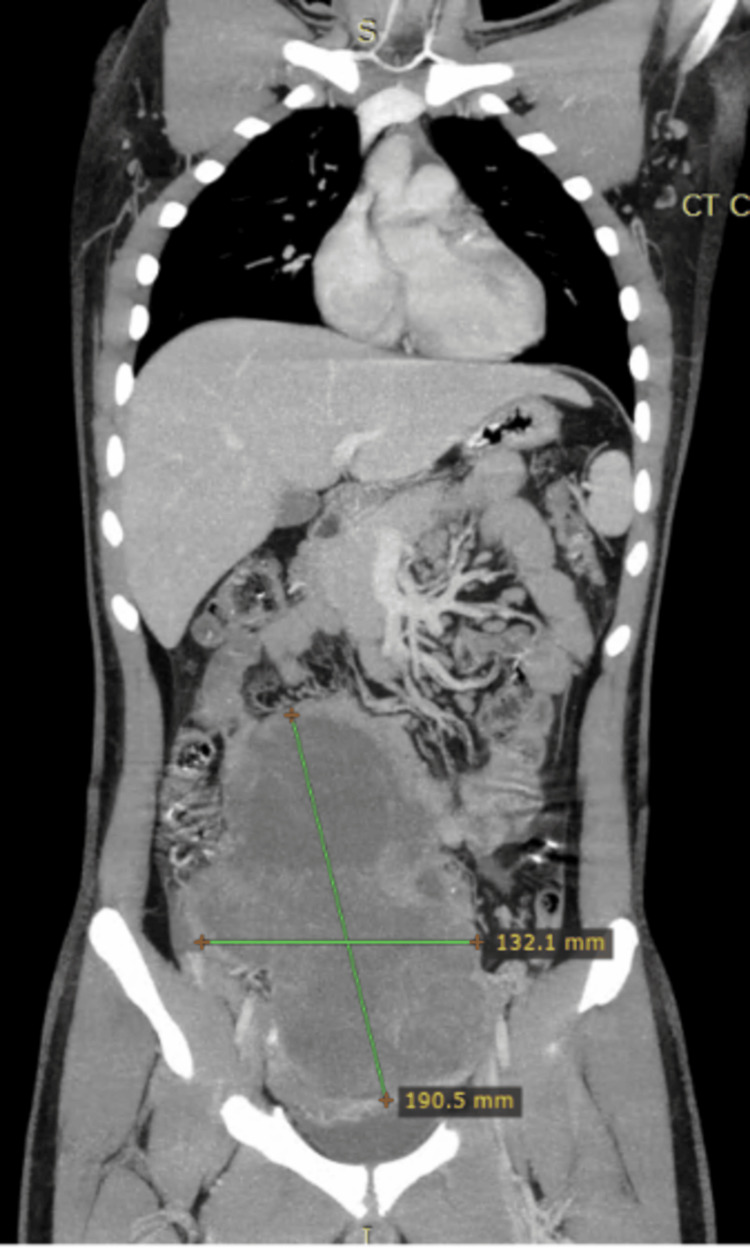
Coronal image of the CT of the abdomen and pelvis showing a mass of around 19×13 cm CT: computed tomography
